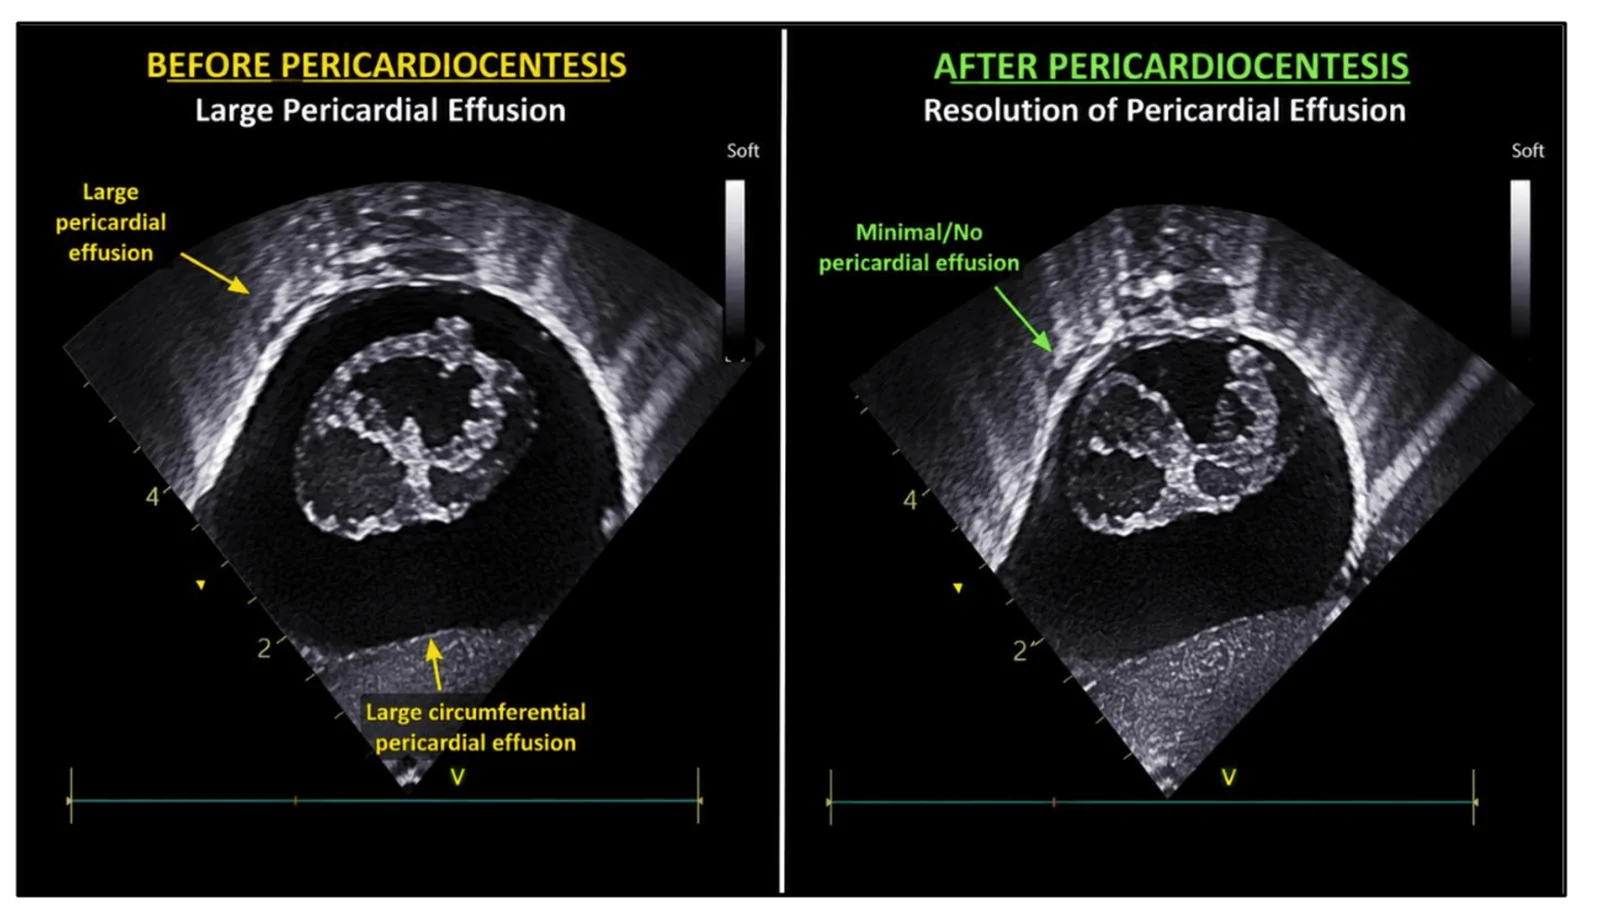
Apical four-chamber echocardiographic images before and after pericardiocentesis showing a large circumferential pericardial effusion with tamponade physiology (left) and near-complete resolution of the effusion following drainage (right)
